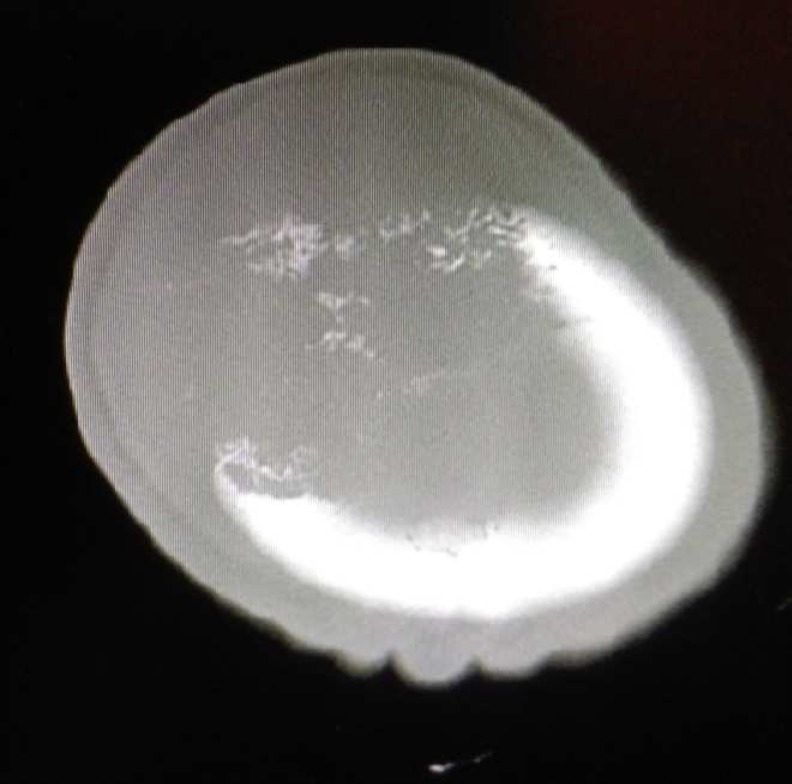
The bone window brain computed tomography (CT-scan)
